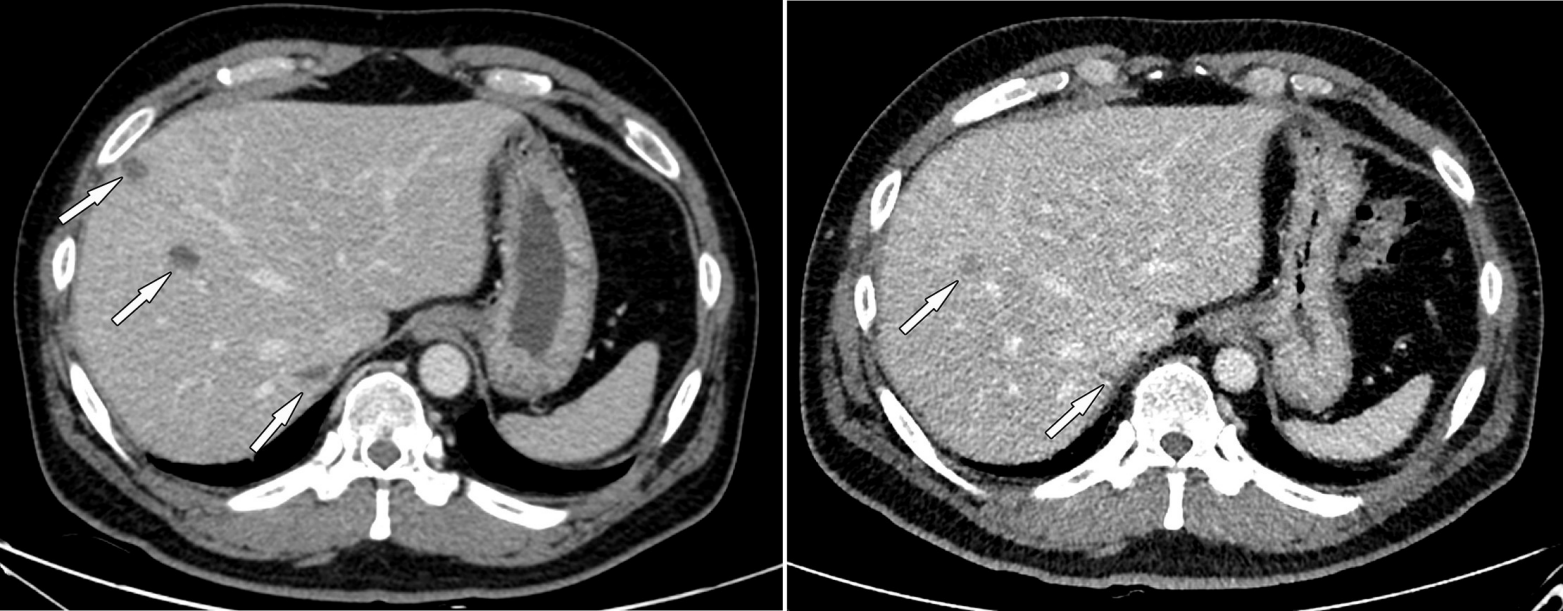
Contrast-enhanced CT images of a 46-year-old man with liver abscesses. Body mass index was 30.7 kg/m^2^. (A) Initial standard-dose CT shows several low attenuation lesions in the right hemiliver (arrows), suggestive of liver abscesses. (B) Follow-up ultralow-dose CT shows the liver abscesses decrease in size (arrows). The CT dose index volume of initial CT and follow-up CT was 15.4 mGy and 3.7 mGy, respectively. Image quality and noise were worse on follow-up CT, but diagnostic confidence was relatively preserved. The scores for image quality, noise, and diagnostic confidence were 2, 1, and 5 for reader 1, and 1, 1, and 4 for reader 2.
